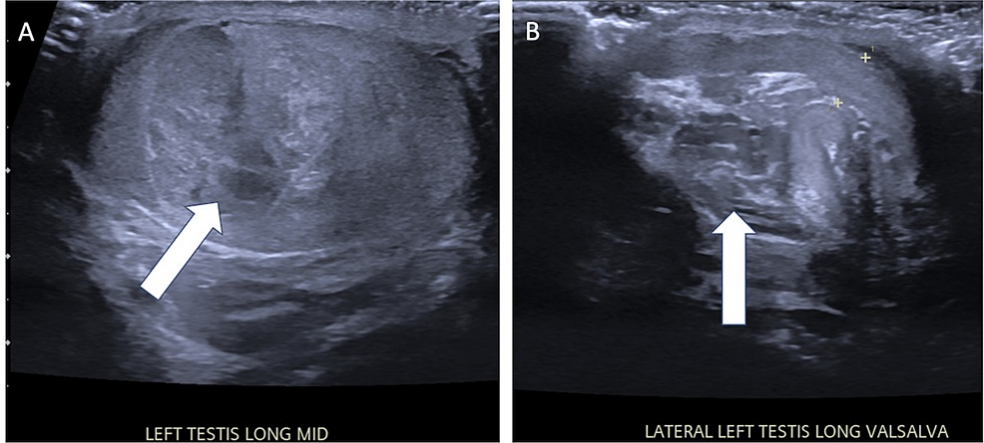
Left testis ultrasound Ultrasound of the left testis demonstrating a mass and associated tortuous vessels (arrows), which are commonly seen in thromboangiitis obliterans (Buerger's disease).
